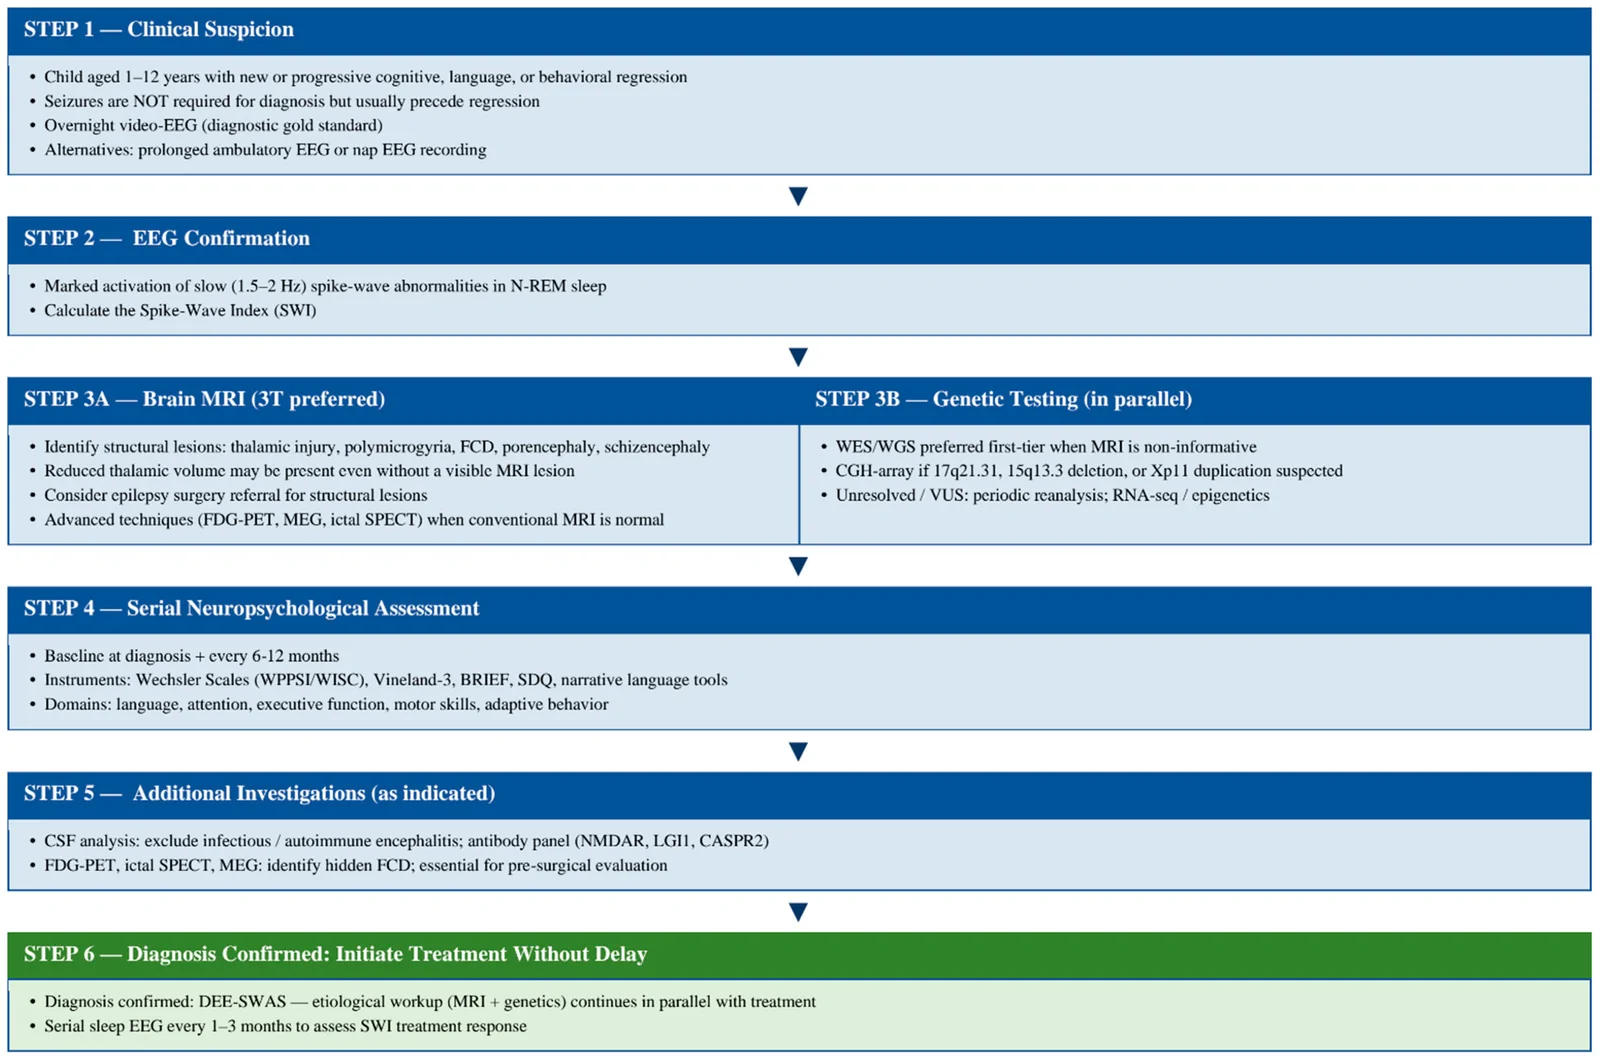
Recommended Diagnostic Algorithm for DEE-SWAS. Abbreviations: CGH = comparative genomic hybridization; CSF = cerebrospinal fluid; EEG = electroencephalogram; FCD = focal cortical dysplasia; FDG-PET = fluorodeoxyglucose PET; MEG = magnetoencephalography; N-REM = non-rapid eye movement; SWI = spike-wave index; VUS = variant of uncertain significance; WES/WGS = whole exome/genome sequencing.
